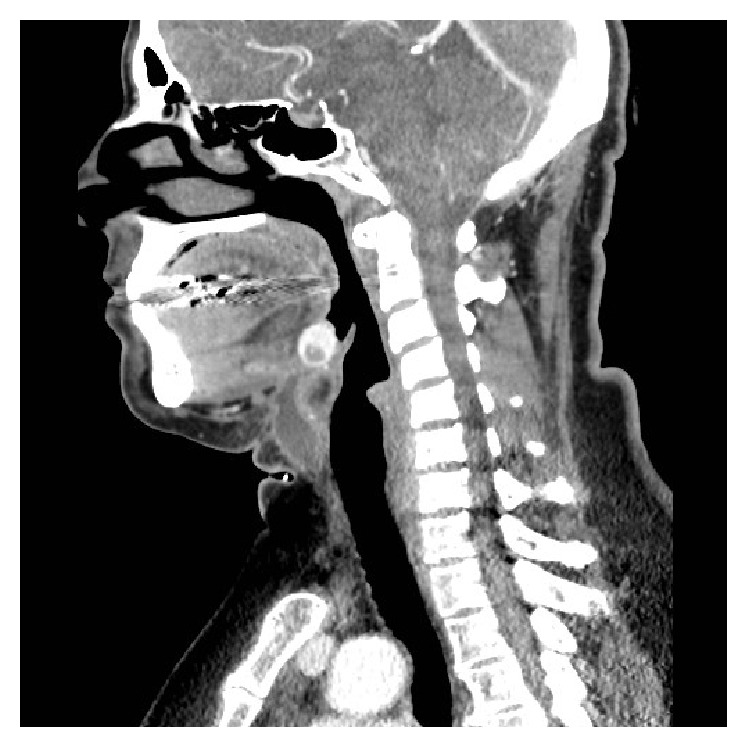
Sagittal CT neck revealing a lingual thyroid without a thyroid in its native position.
